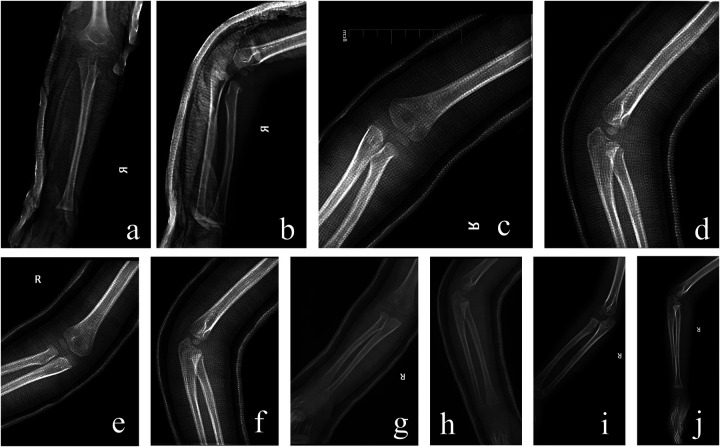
(a,b) Preoperative lateral x-ray shows left radial neck fracture; (c,d) Postoperative anteroposterior and lateral x-ray showed good reduction of the fracture; (e–j). Postoperative x-ray examinations at 1 month, 3 months, and 6 months showed good alignment and callus growth in both the anterior and lateral positions.
